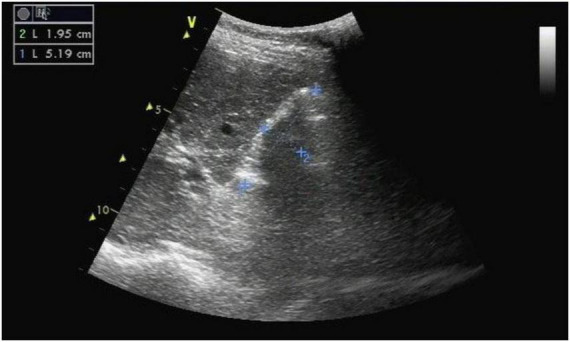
The B-ultrasound revealed that the normal gallbladder structure was not detected in the gallbladder area, and it was replaced by a strong echogenic mass with a range of approximately 5.19 cm × 1.95 cm.
